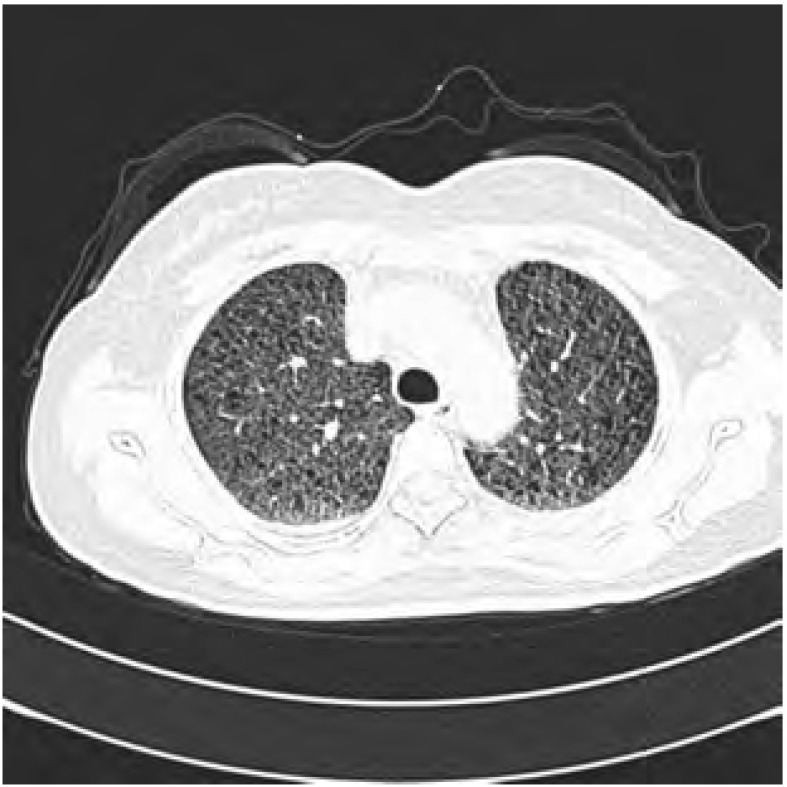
肺HRCT示双肺纹理增多，广泛分布大小不一的含气囊腔 High-resolution CT shows numerous small and sizable cysts distributed diffusely in both lung
